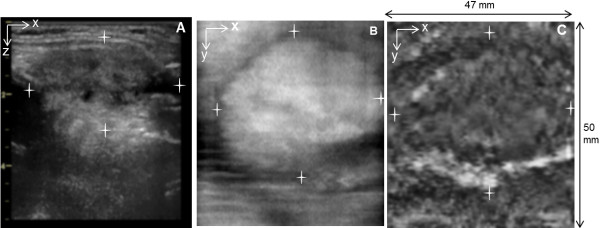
Benign Thyroid Nodule. A) B-mode US of a patient’s thyroid shows a large solid nodule measuring 47×27 mm in the *x*-*z* dimensions on left lobe with a small cystic component, B) Ultrasound C-scan at 2.0 cm depth measuring about 44.2 mm x 30.5 mm in the x-y dimensions, and C) *In vivo* VA image of thyroid (with 7 L array probe, *f*_1_ = 3.64 MHz and Δ*f* = 54.27 kHz) at 2.0 cm depth which correlates to the one of C-scan plane, measuring more than 47 mm in the *x*-direction and 30.5 mm in the *y*-direction and the star shows the dimensions. The VA image is 47 ×50 mm.
